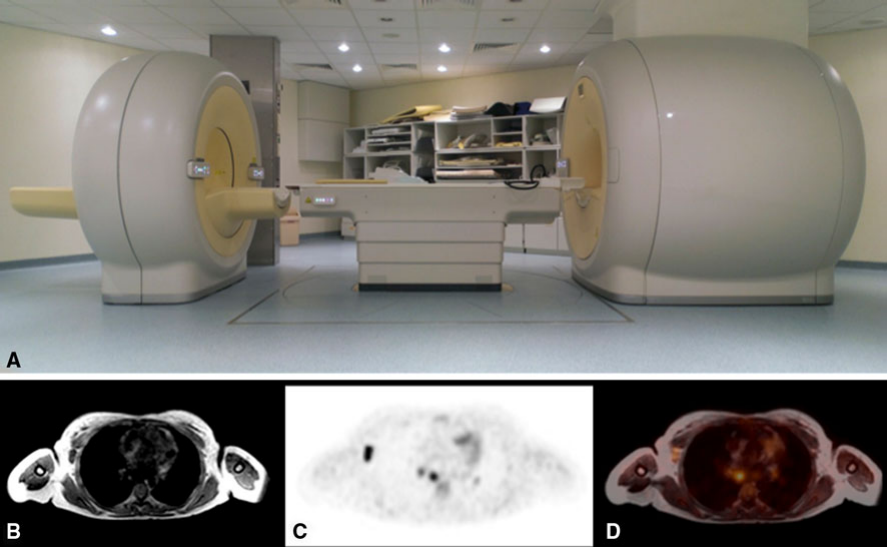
a Whole-body PET/MR system; b attenuation MR, a low-resolution gradient echo scan; c FDG-PET scan; d fused PET/MR image
